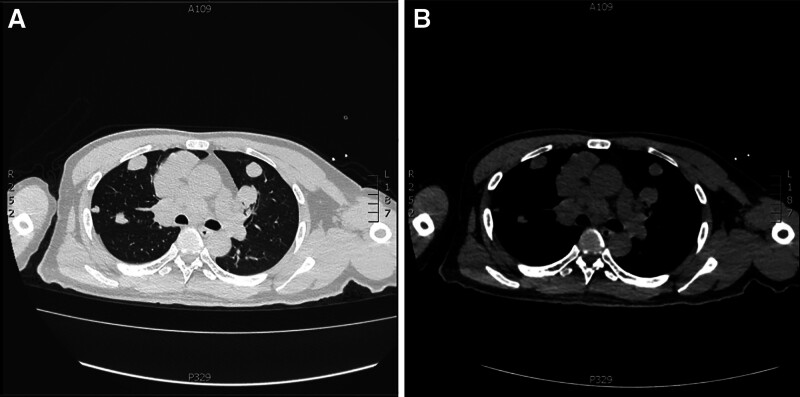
Patient chest CT examination results: (A) the lung window shows diffuse soft tissue lesions in both lungs combined with multiple enlarged lymph nodes in the mediastinum, some of which are fused, suggesting metastatic tumors (lung window); (B) in the mediastinal window at the same level, the lesions appear as solid soft tissue density. CT = computed tomography.
